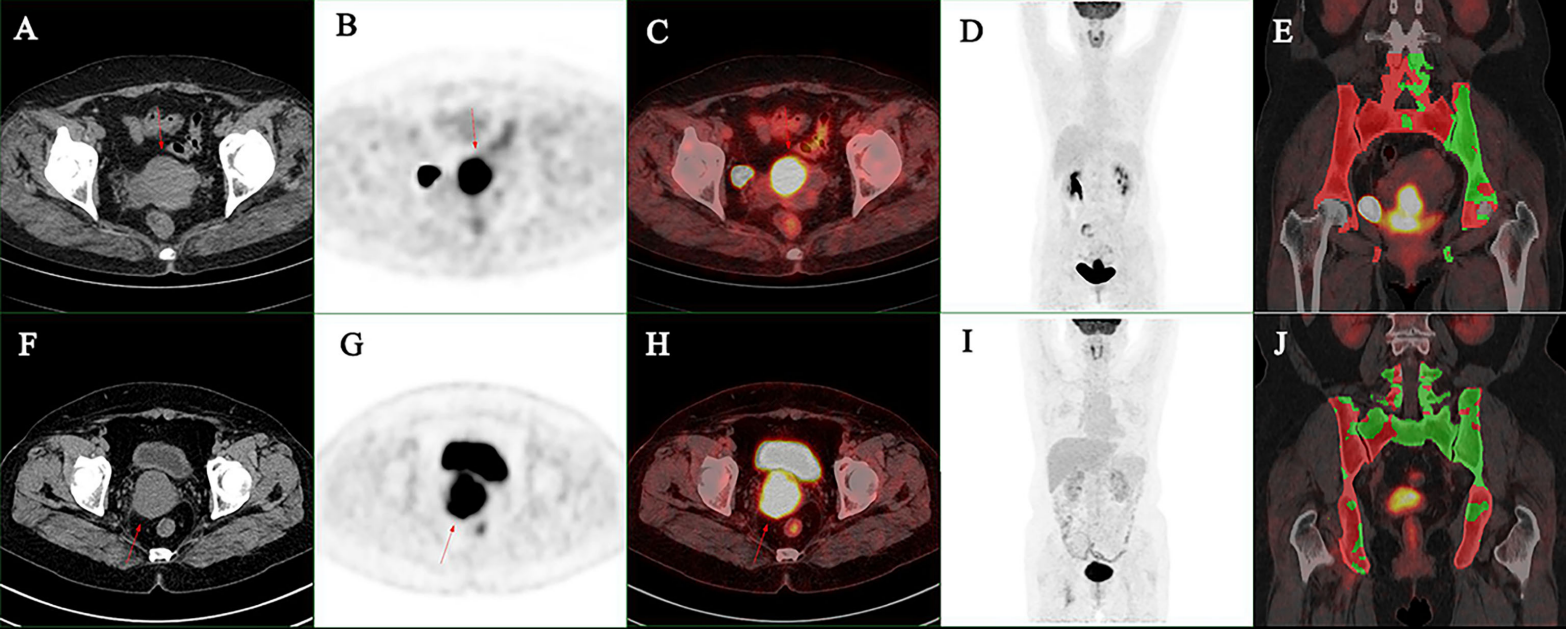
(A–E) A 47-year-old women suffered FIGO stage IIIC1r cervical cancer, the maximum diameter of primary lesion was 6.0 cm: the SUVmax was 17.5, the MTV was 39.4 cm^3^, and the BM_ACT_% was 31.2%. G3+ hematologic toxicity occurred during chemoradiotherapy. (F–J) A 55-year-old women suffered FIGO stage IIIC1r cervical cancer: the maximum diameter of primary lesion was 5.1 cm, the SUVmax was 12.9, the MTV was 39.5 cm^3^, and the BM_ACT_% was 49.8%. G3+ hematologic toxicity occurred during chemoradiotherapy. (A, F) Axial CT image of primary lesion. (B, G) Axial PET image of primary lesion. (C, H) Axial PET/CT fusion image of primary lesion. (D, I) Maximum intensity projection of trunk PET. (E, J) Coronal PET/CT fusion image with BM_ACT_ shows in green.
